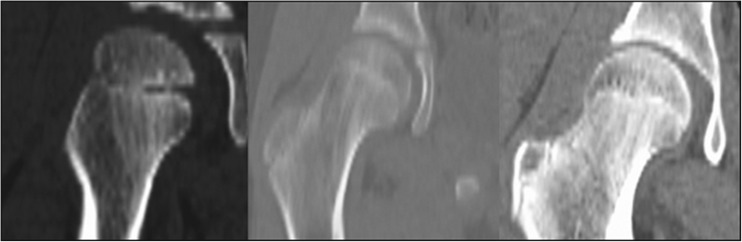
CT scan of a growth plate of the proximal femoral epiphysis in a 7-, 10- and 13-year-old child
